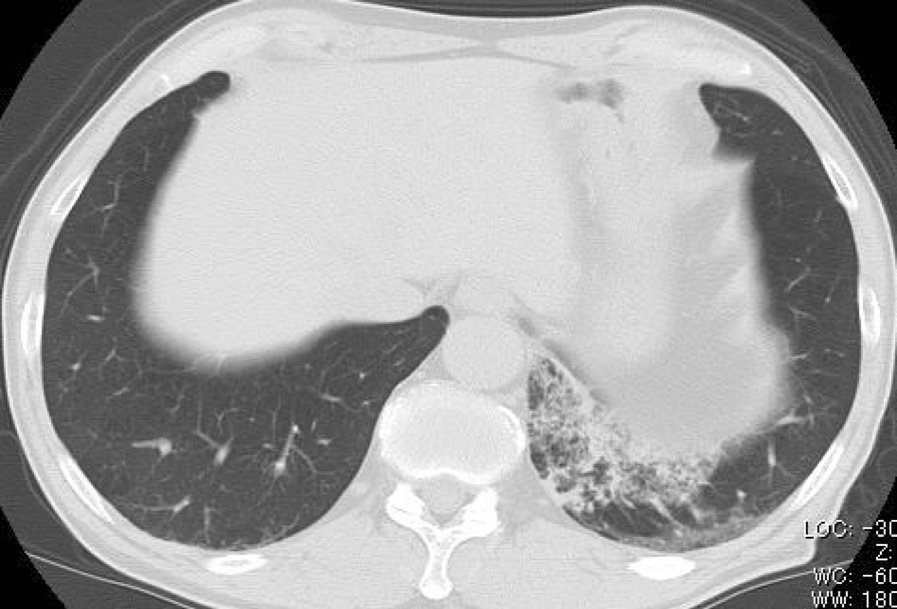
Chest computed tomographic scan shows diffuse pneumonia-like shadow in the left S^10 ^segment.
